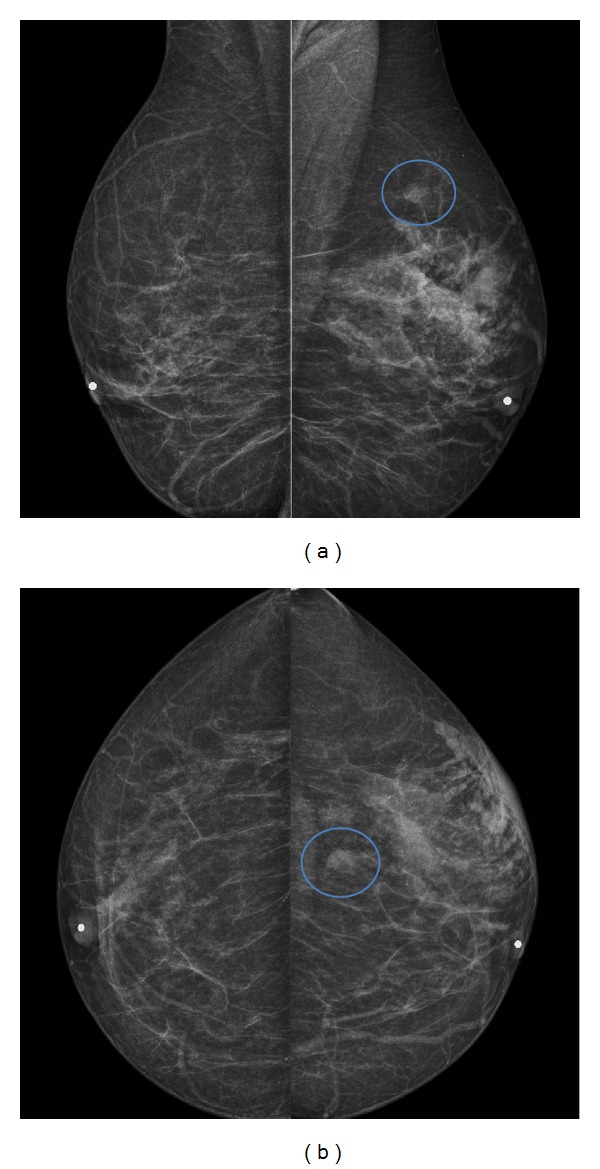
Bilateral mammography*: bilateral mediolateral oblique (a) and craniocaudal (b) mammographic views demonstrate scattered fibroglandular tissue with global asymmetry and focal skin thickening in the left breast which was classified as moderate suspicious BI-RADS 4c. There was also an isodense, round, circumscribed mass, measuring 12 × 8 mm in the outer upper quadrant, requiring complementary ultrasonography (encircled). Nipples are marked.
